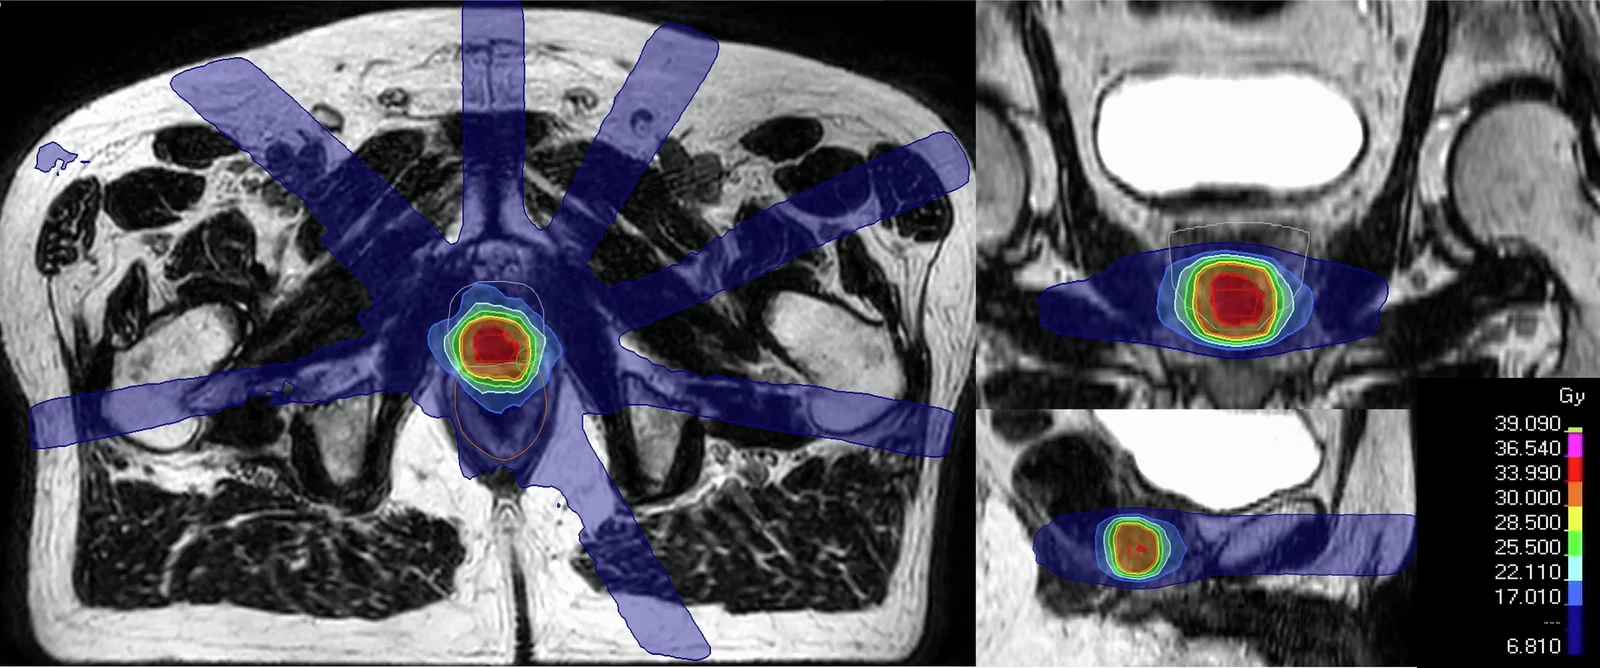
An example of a clinically delivered plan. Axial and coronal views on planning MRI to show focal GTV, prostate gland contoured in light gray and dose colourwash, with Gy shown in the legend.
